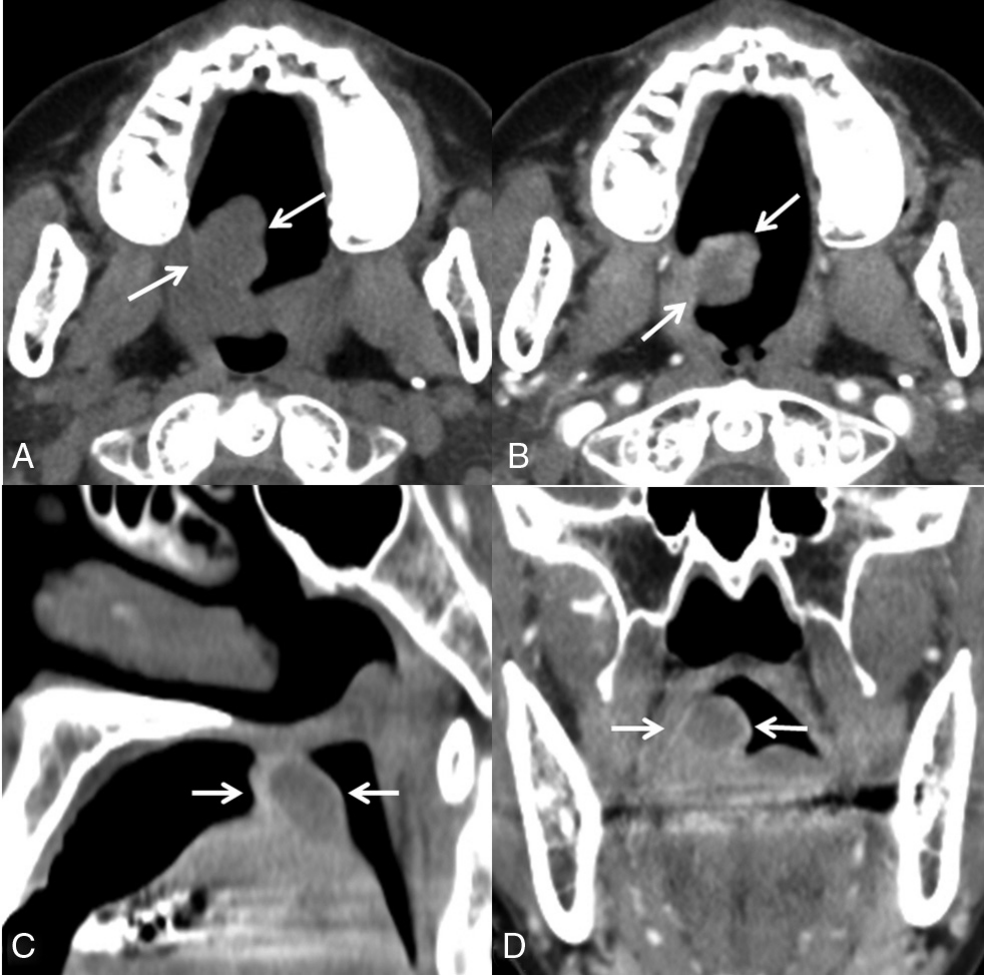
(A) Axial pre-contrast computed tomography (CT) showed a well-delineated homogeneous mass without chondroid matrix calcification. (B–D) Contrast-enhanced CT showing showed a peripheral enhancing mass with internal heterogeneous enhancing portion and the size was measured to be approximately 3.0 cm × 2.0 cm × 1.6 cm. The soft tissue mass was separated from the bone.
